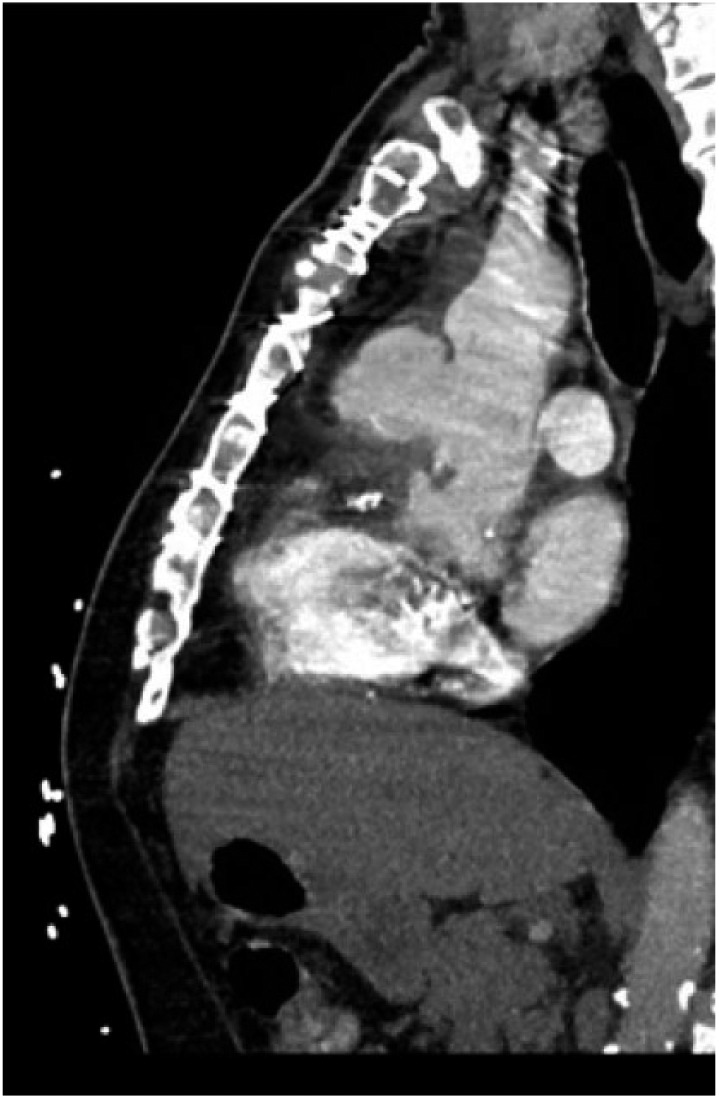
Initial CT angiogram of the chest with aortic pseudoaneurysm.
